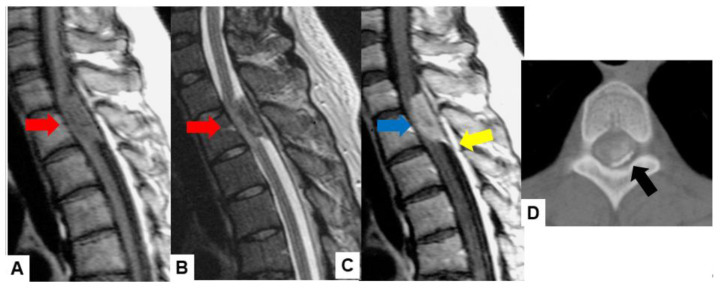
Forty-two F, spinal meningioma. (A) T1-weighted midsagittal image, (B) T2-weighted midsagittal image, and (C) enhanced T1-weighted midsagittal image. (D) CT. Red arrows indicate tumor and blue arrow shows tumor enhancement. Yellow arrow shows dural tail sign and black arrow indicates tumor ossification.
